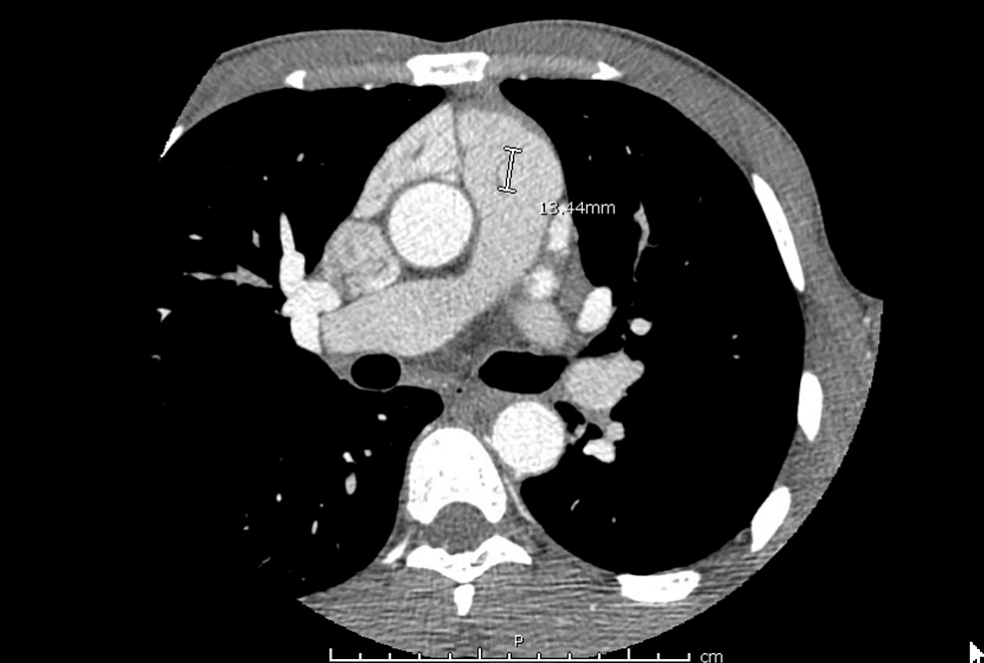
Cardiac computed tomography showing thin, curvilinear strand-like densities surrounding the pulmonic valve consistent with the findings of the prior echocardiogram suggesting vegetation likely resolving
